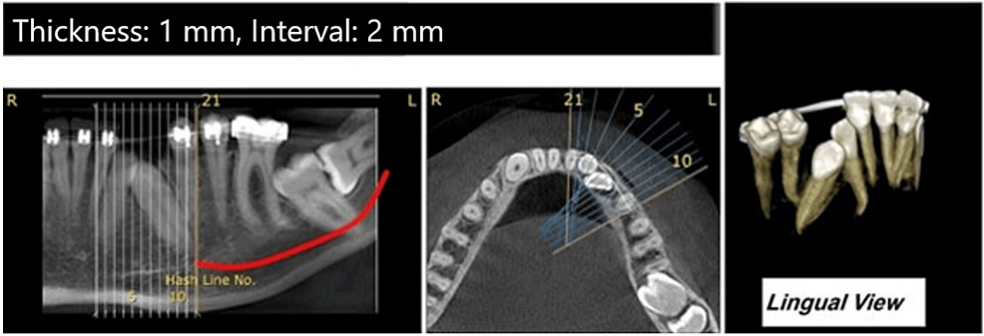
Pretreatment cone-beam CT image of anterior mandibular teeth
